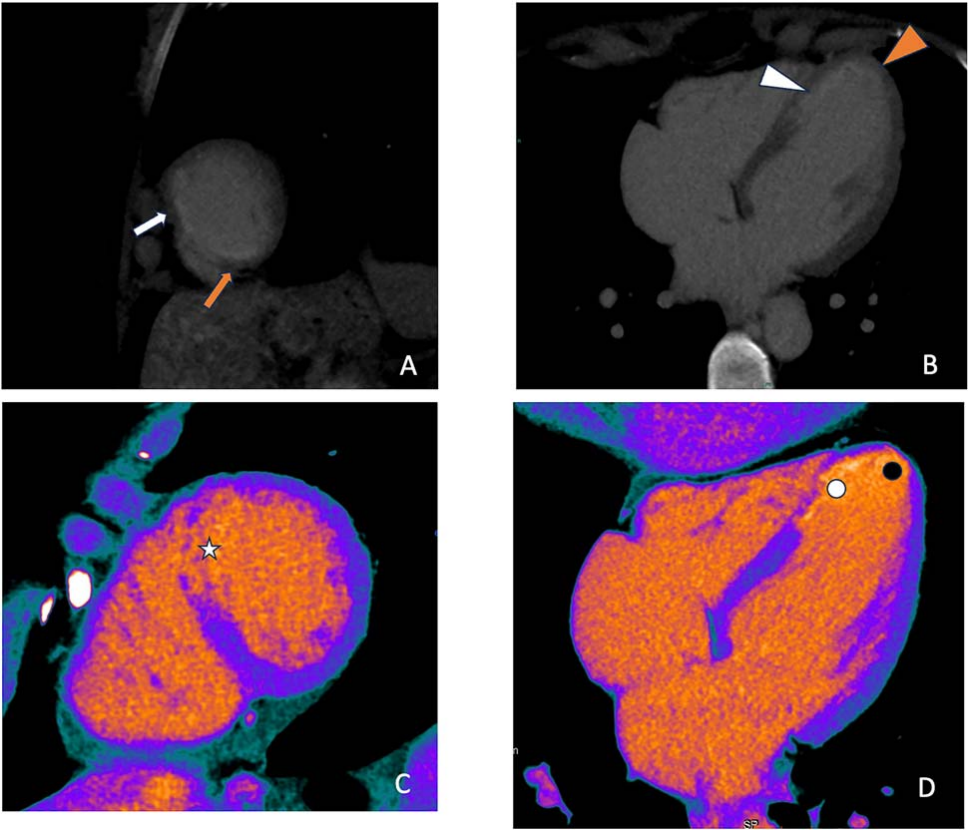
Delayed scan at follow-up CT in august 2023 shows nearly transmural LIE of the mid-septal, apical-septal and apex on short axis (A, white and red arrow) and four chamber (B, white and red arrowheads) reconstructions with MVO no longer appreciable. The same findings are more easily visualized on colorimetric perfusion maps (short axis in C with white star; four-chamber view in D with white and black point).
